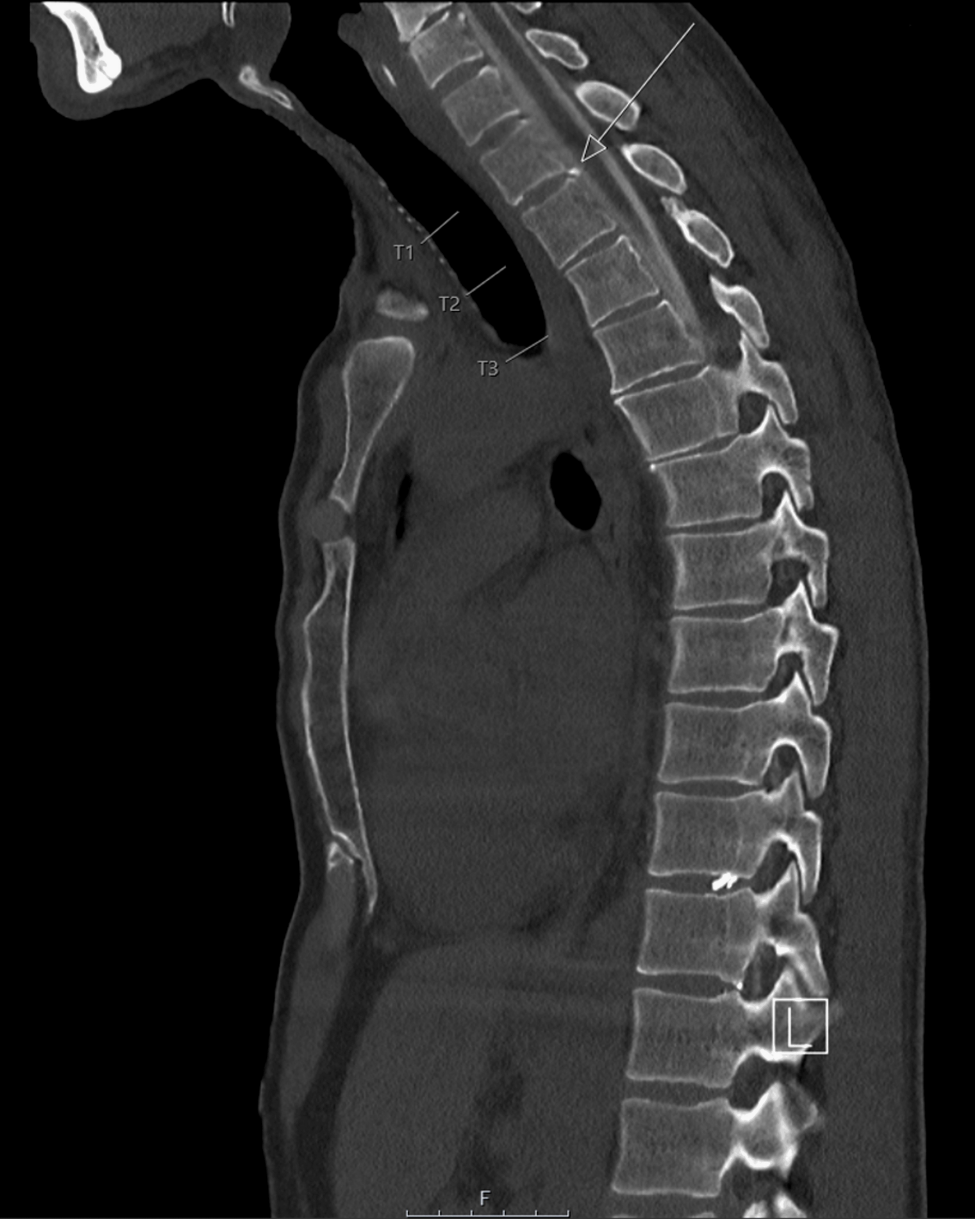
CT spine myelogram showing an arrow pointing to a small calcified, pointed osteophyte at the T1-T2 level
